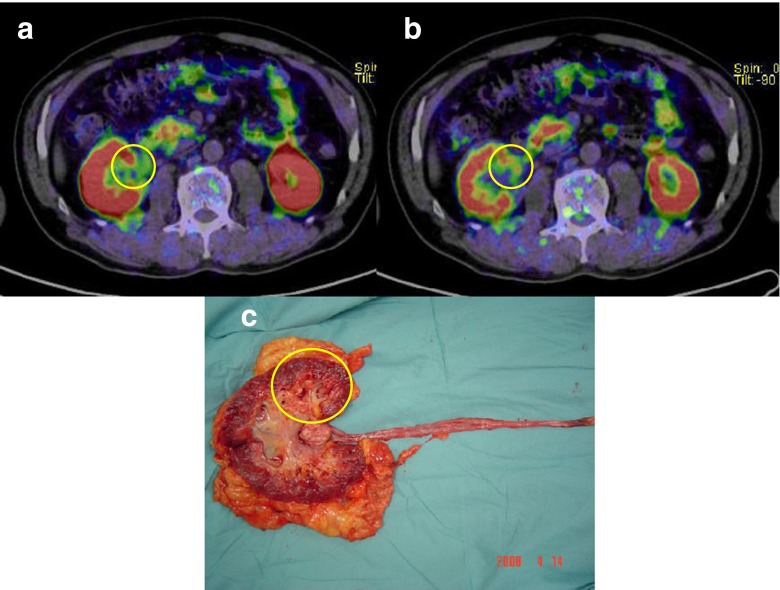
Urothelial carcinoma of the left renal pelvis in patient 1. a, b Choline PET/CT images of the primary tumour at 10 min (a) and 20 min (b) after injection. Choline uptake at 10 min is positive but weak (SUVmax 2.48) compared with that at 20 min (SUVmax 3.79). c Photograph of the primary tumour after resection. *Circles* tumour sites
